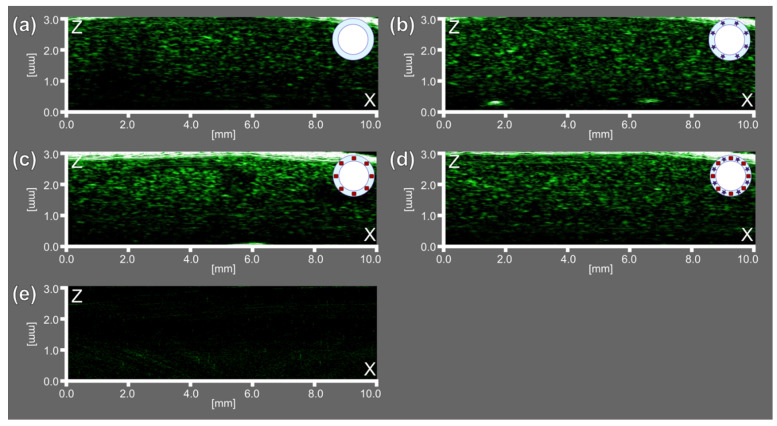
Ultrasound (US) imaging at a frequency of 33 MHz for (a) BSA MBs, (b) BSA-ZnPc MBs, (c) BSA-Au MBs, (d) BSA-Au-ZnPc MBs, and (e) saline solution.
